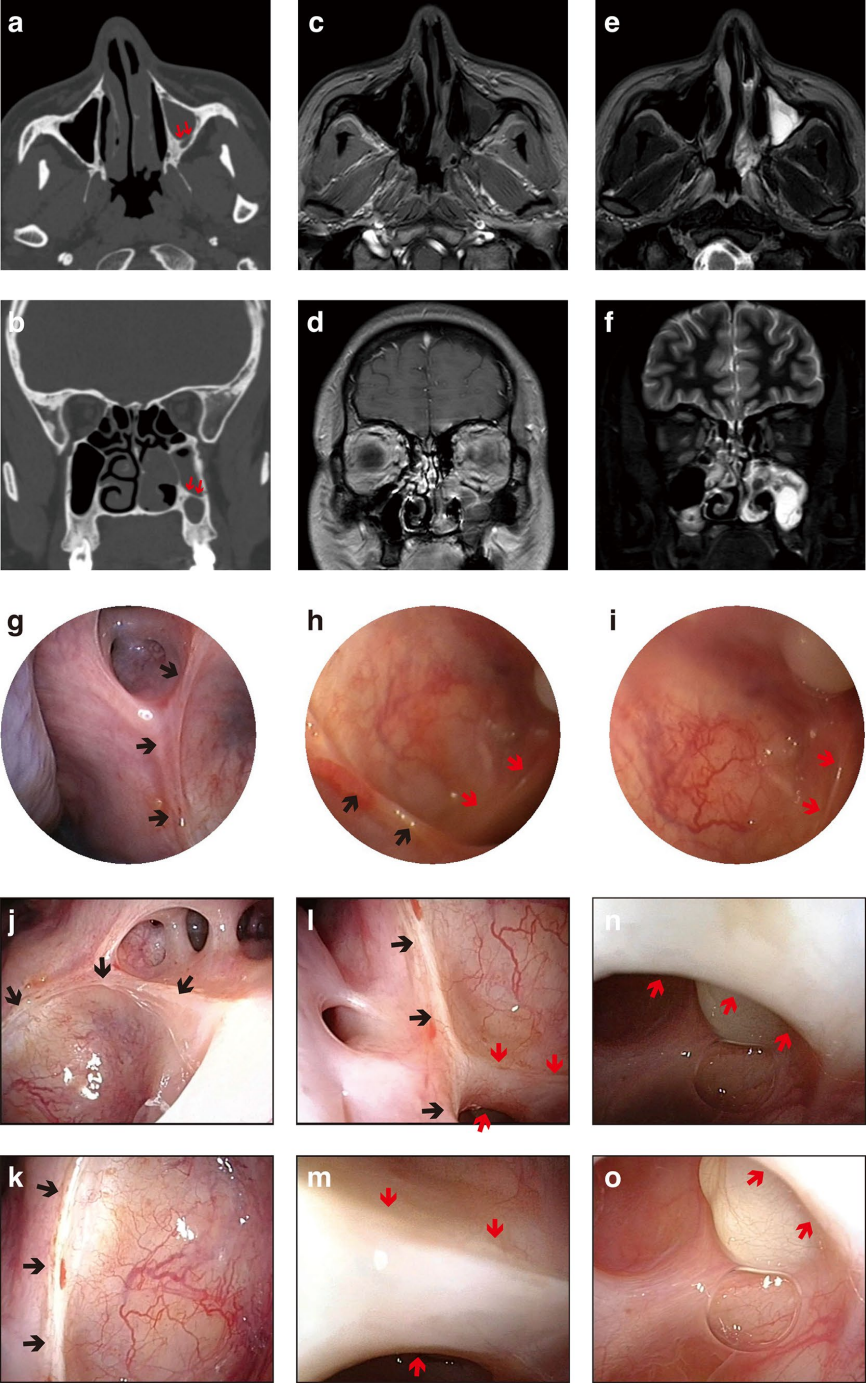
Bone septum on the inferior wall of MS bypassed via flexible endoscope in Case 1. A–f Preoperative medical images of the patient, including CT images (a, b), T1 weighted contrast-enhanced MRI (c, d) and T2 weighted MRI (e, f). A bone septum (red arrows) was revealed clearly on CT image. g–i Inner surfaces of MS were observed by endoscopes through ostium (black arrows). Postoperative follow-up of MS using 0° (g) and 70° (h, i) rigid endoscopes. j–o The bone septum (red arrows) could be bypassed using flexible endoscope (Color figure online)
